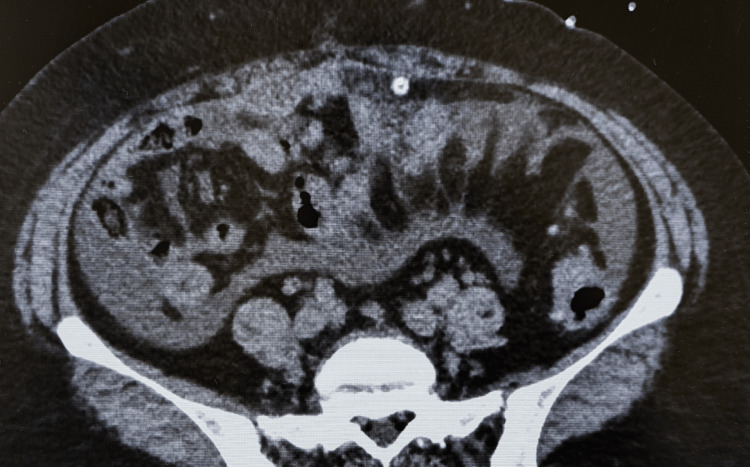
Computed tomography findings There are no notable abnormalities except for the presence of the PD catheter. PD: peritoneal dialysis
